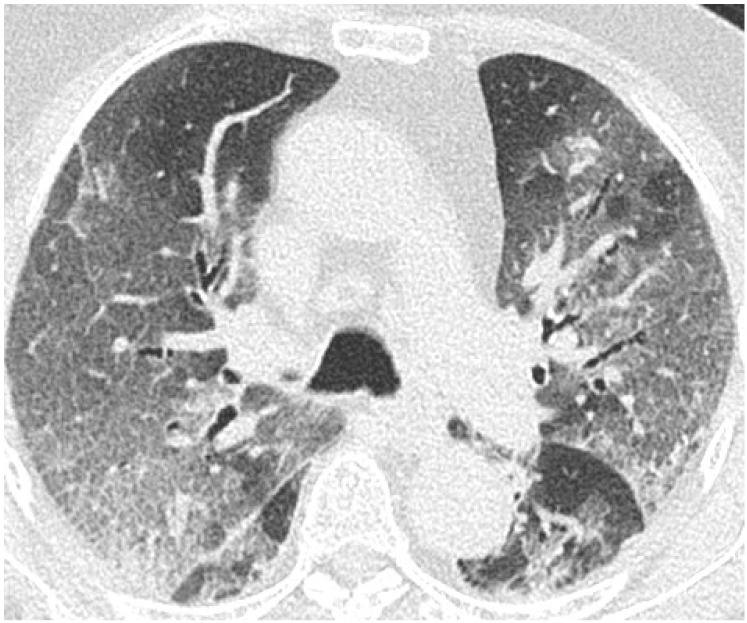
Axial plane of non-enhanced CT reveals bilateral ground glass opacities with superimposition of interlobular septal thickening, giving the crazy paving appearance, distributed symmetrically in both lungs.
